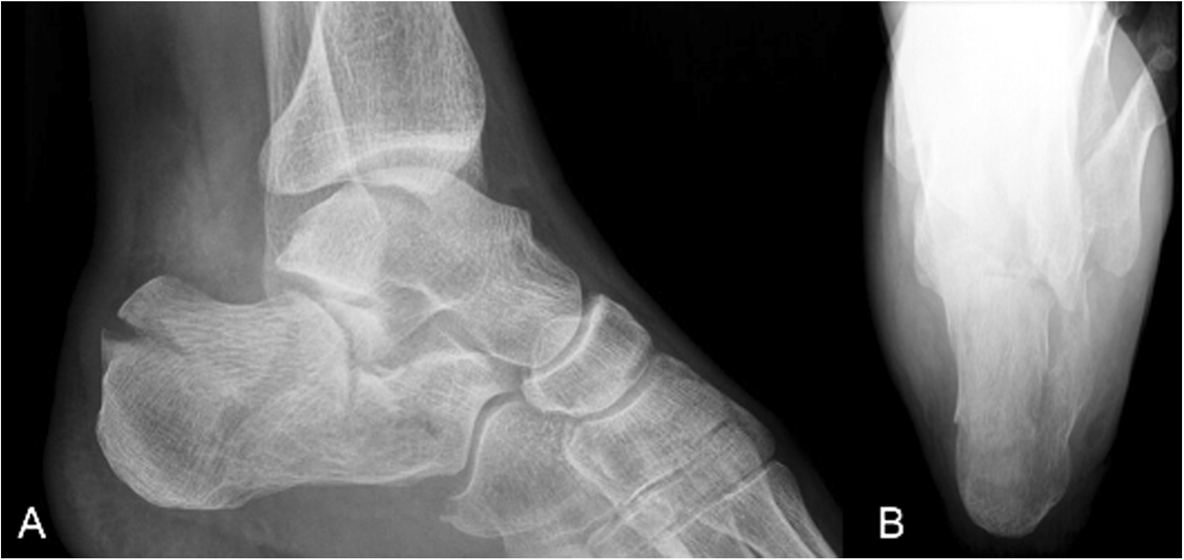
Case 1 Preoperative radiographs. Radiographs left calcaneus lateral (A) and axial (B) view, 62 year old male patient, non-smoker
